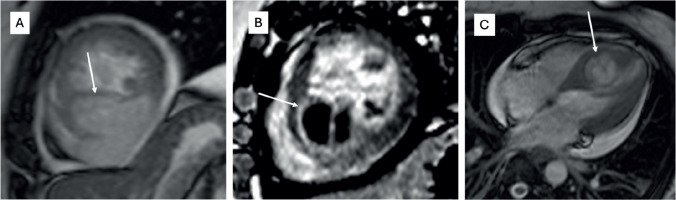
Cardiac MRI of a patient with synovial sarcoma of interventricular septum – (a): Bright blood (SSFP sequence) in short-axis view showing the heterogeneously hyperintense mass lesion (arrow) involving the septum and extending into the inferior wall of the left ventricle; (b): Late gadolinium enhancement (LGE) sequence in short axis view showing heterogenous peripheral enhancement with central non-enhancing component; (c): Bright blood (SSFP sequence) in four chamber view showing the mass (arrow) with cystic appearance within it.
